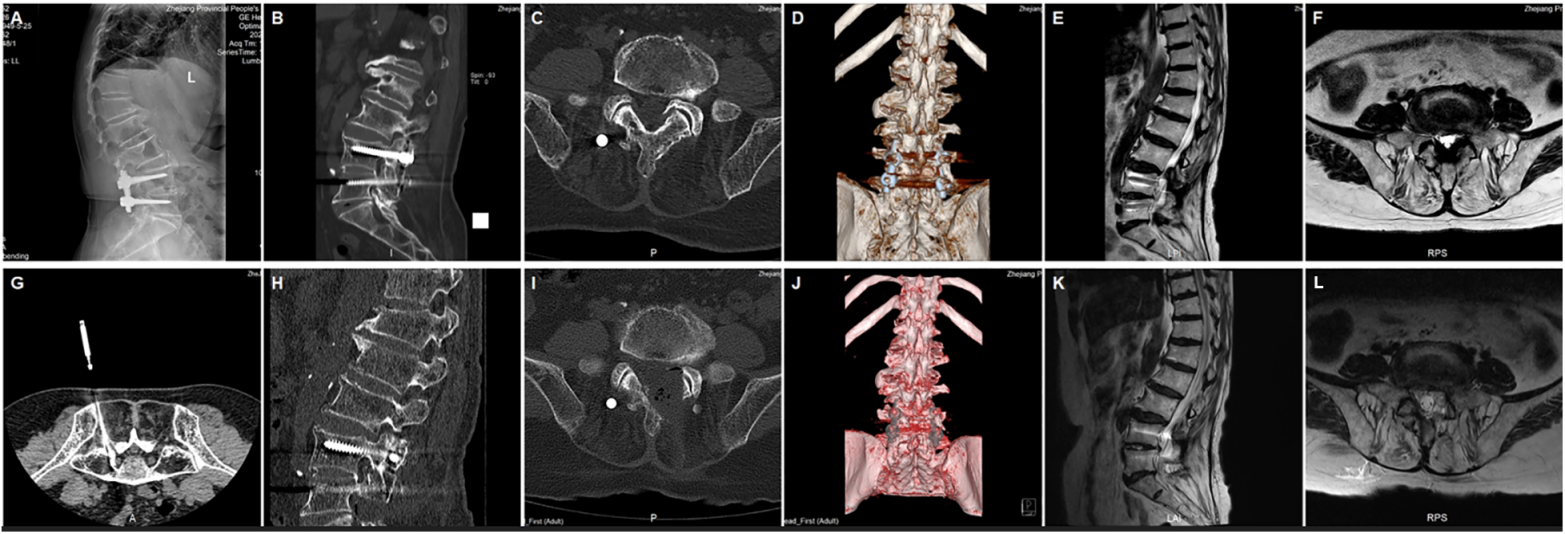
(A) Preoperative x-ray displaying spinal fusion (B) CT showing hyperplasia of small joints (C) CT showing stenosis of the lateral recess (D) preoperative CT three-dimensional reconstruction (E) preoperative T2-weighted MRI axial plane display of spinal canal stenosis (F) preoperative T2-weighted MRI sagittal plane display of spinal canal stenosis (G) Pre-surgery CT-guided selective nerve root block tests to pinpoint the responsible segment (H) CT showing removal of the small joint (I) CT showing removal of the left inferior lamina (J) CT three-dimensional reconstruction displaying inner edge small joint and partial removal of the vertebral plate (K) postoperative T2-weighted MRI sagittal plane (L) postoperative T2-weighted MRI axial plane.
